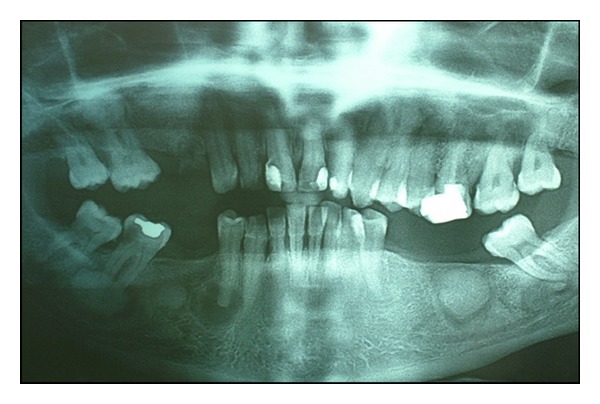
The first orthopantomography revealed the presence of three well-circumscribed, round, unilocular neoformations of radiopaque appearance with a radiotransparent edge, one of which was in close contact with the roots of the lower right second molar.
